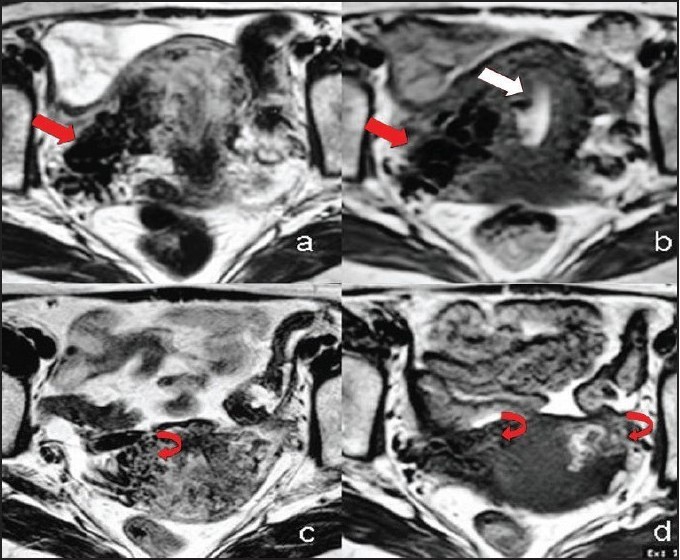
Pre-embolisation Axial T2W (a) and T1W (b) MRI scans at the level of uterine body and cervix show presence of multiple tortuous flow voids suggesting abnormal vessels in the myometrium and adenexa (solid arrows). More number of abnormal vessels is seen on the right side. Also blood (hollow arrow) is seen in the endometrial cavity (b). Comparable post-embolisation Axial T2W (c) and T1W (d) MRI scans show thrombosed vessels (curved arrows)
